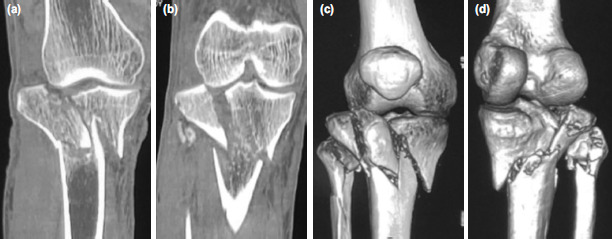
Case No.12, pre-operative CT images. (a) Coronal section image showing Shatzker Type VI fracture. (b) Coronal section image showing tibial condylar widening and intra-articular step. (c) 3D reconstruction view anterior aspect. (d) 3D reconstruction view posterior aspect.
